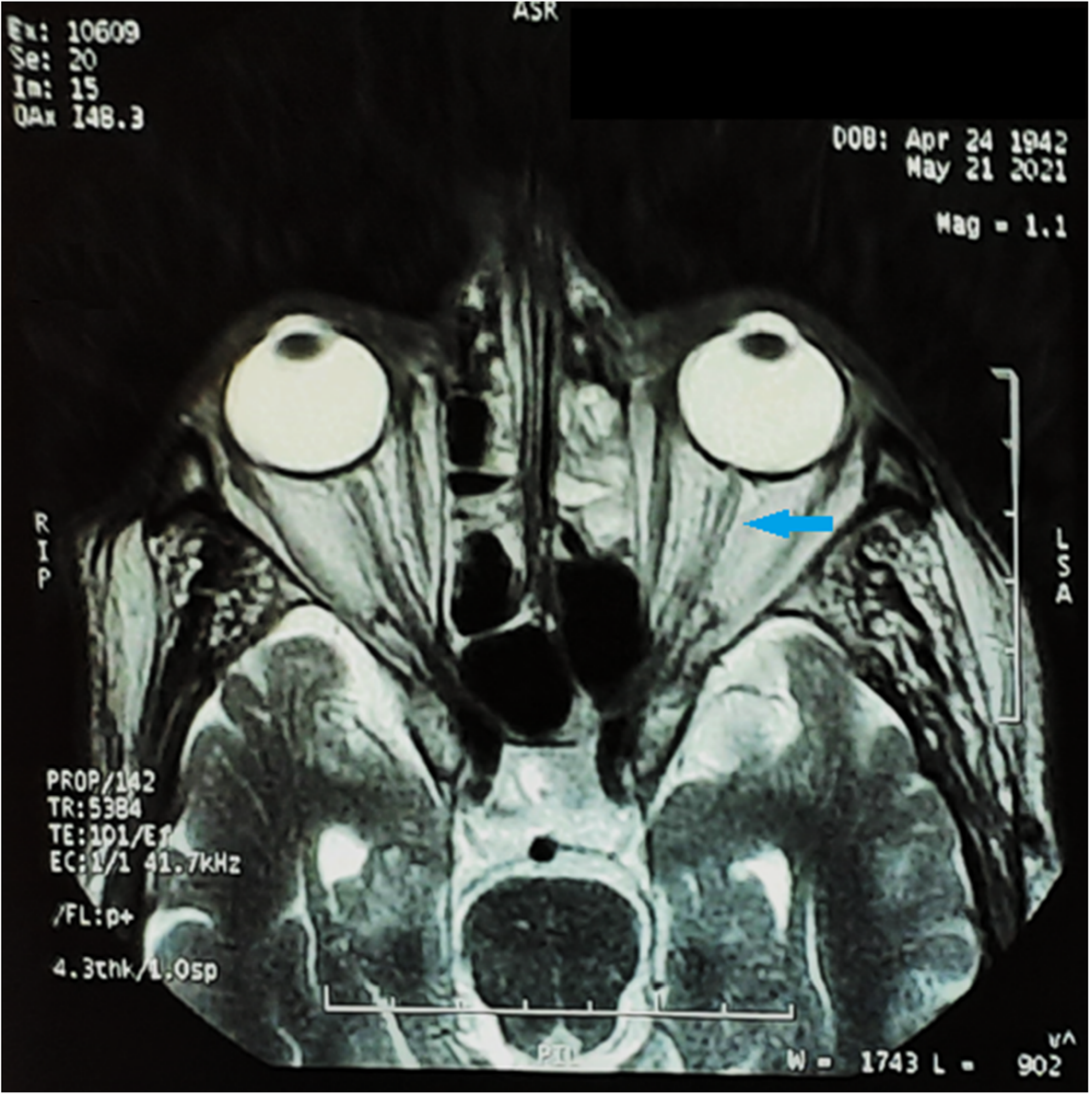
Blue arrow along non contrast MRI T2 WIs highlighting mild form of left optic neuritis
